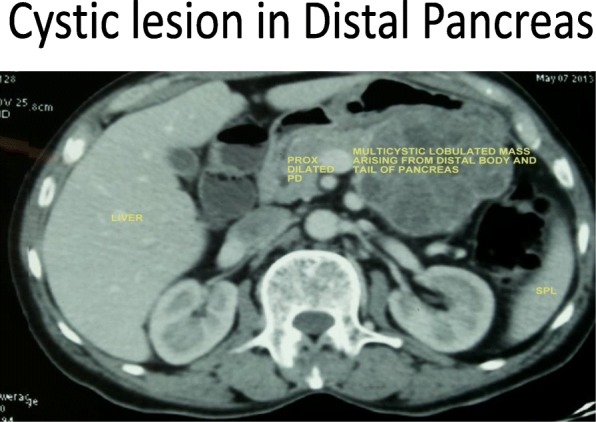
Cystic lesion in distal pancreas on computed tomography
